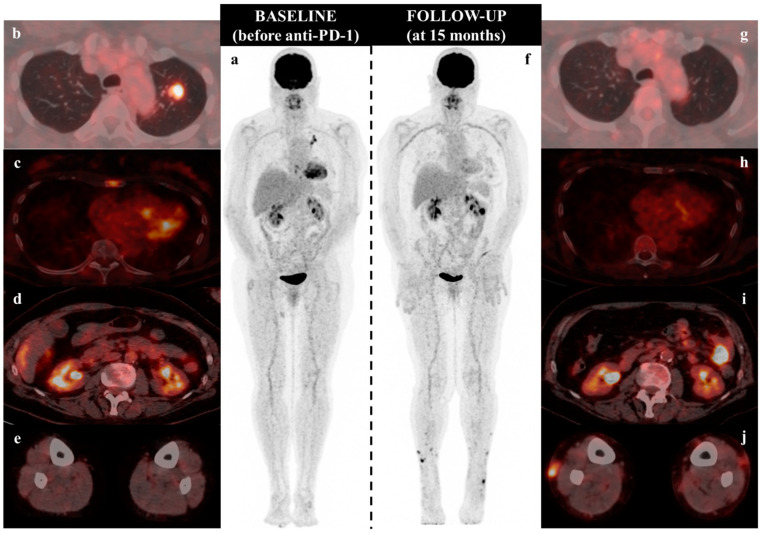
[18F]-FDG PET/CT scans with MIP (maximum intensity projection: (a,f) and fused axial images at the initiation of PD-1 inhibitor as single-agent and follow-up (15 months). The injected dose of FDG and the time of acquisition stays comparable across examinations and the maximum standardized uptake value (SUVmax) was used for measuring the uptake of FDG by pathological tissue. The patient received Nivolumab 240 mg every two weeks for the first six cycles and then 480 mg every four weeks, without any dose reduction. Assessment of response to immunotherapy was performed using CT scans every 2–3 months. While a partial morphologic response had been obtained at two months, the best overall response was achieved at seven months with a complete response. At 10 months, very small skin lesions occurred on the legs, and the radiologic presentation strongly suggested progressive disease at 14 months with the appearance of a mesenteric lymph node metastasis. [18F]-FDG PET/CT scan was thus performed for restaging, which confirmed the complete metabolic response in the upper lobe of the left lung (b) SUVmax 9.0 and (g) no pathologic uptake and in the sternal bone (c) SUVmax 5.5 and (h) no pathologic uptake. Moreover, we confirmed the presence of a mesenteric lymph node (d) no pathologic uptake and (i) SUVmax 13.1, sticking to the colonic wall, which explains why it can hardly be individualized. PET/CT exam also revealed the appearance of several FDG-avid subcutaneous nodules on her legs (e) no pathologic uptake and (j) SUVmax 10.5 on the left leg and 10.2 on the right leg. Such findings have been reported in patients with non-small cell lung cancer [1], esophageal cancer [2], and ocular melanoma [3] treated by PD-1 inhibitors. Similar images have also been published in metastatic cutaneous melanoma treated by BRAF and MEK inhibitors [4]. Recently, Tetzlaff et al. reported 3 patients with advanced cutaneous melanoma treated by ICIs who developed granulomatous/sarcoid-like lesions (2 with an antibody targeting PD-1 and 1 with an antibody targeting CTLA-4) [5]. However, only one patient presented multiple subcutaneous nodules suggesting erythema nodosum, with a very different presentation from our patient. First, this patient developed subcutaneous lesions on his bilateral dorsal hands, forearms, and elbows, but not on his legs, as we described for our patient. Second, the patient developed concurrently other granulomatous/sarcoid-like lesions, as well as new hypermetabolic hilar and mediastinal lymph nodes, while for our patient, subcutaneous nodules on legs were isolated.
